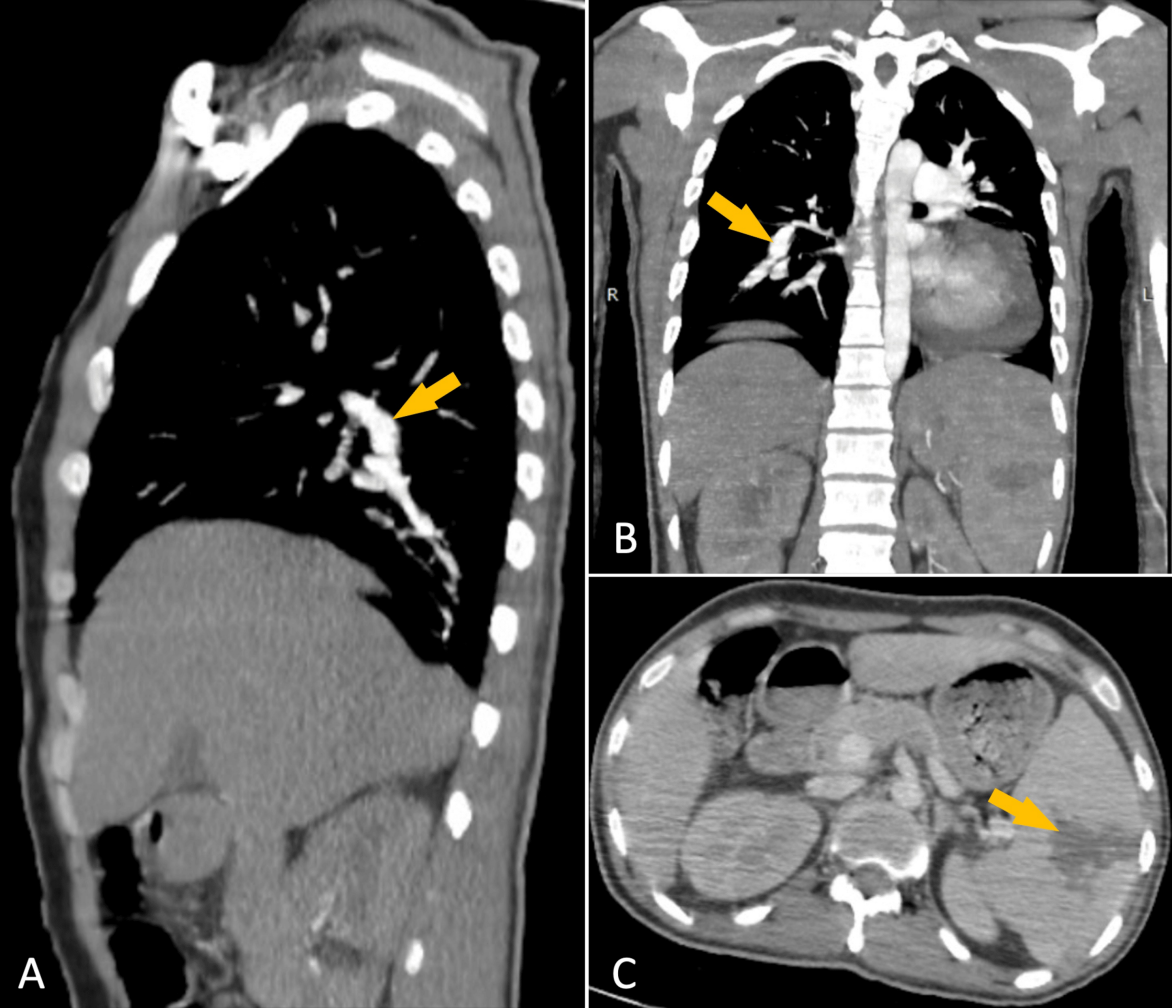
Thoracic and abdominal CT scan. Sagittal (A) and coronal (B) views reveal a mycotic aneurysm of the superior segmental artery of the right upper lobe (arrows). A transverse section (C) demonstrates a splenic infarction.
